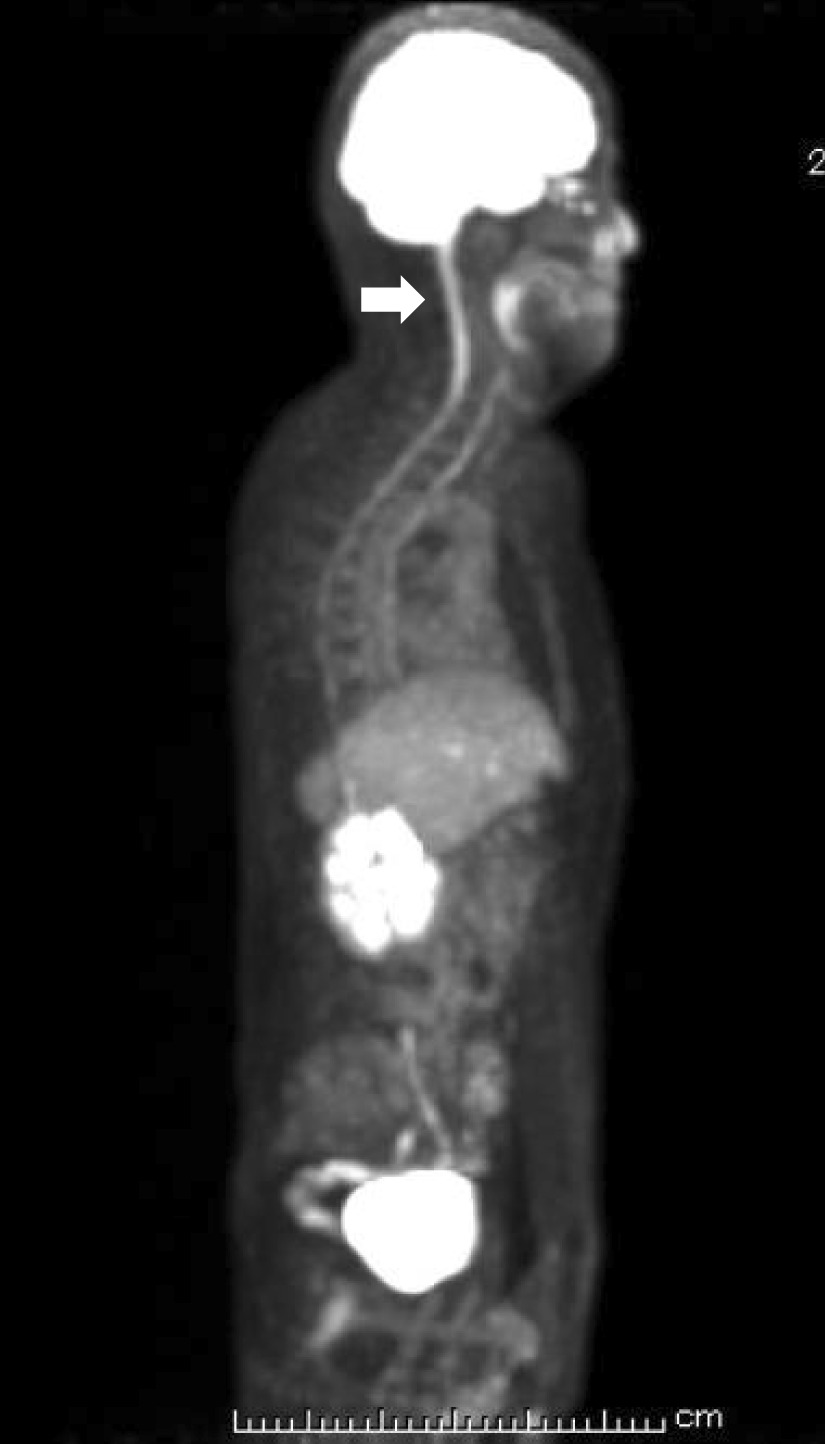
18F-FDG PET-CT imaging. The arrow indicates the abnormal accumulation of FDG along cervical cord
